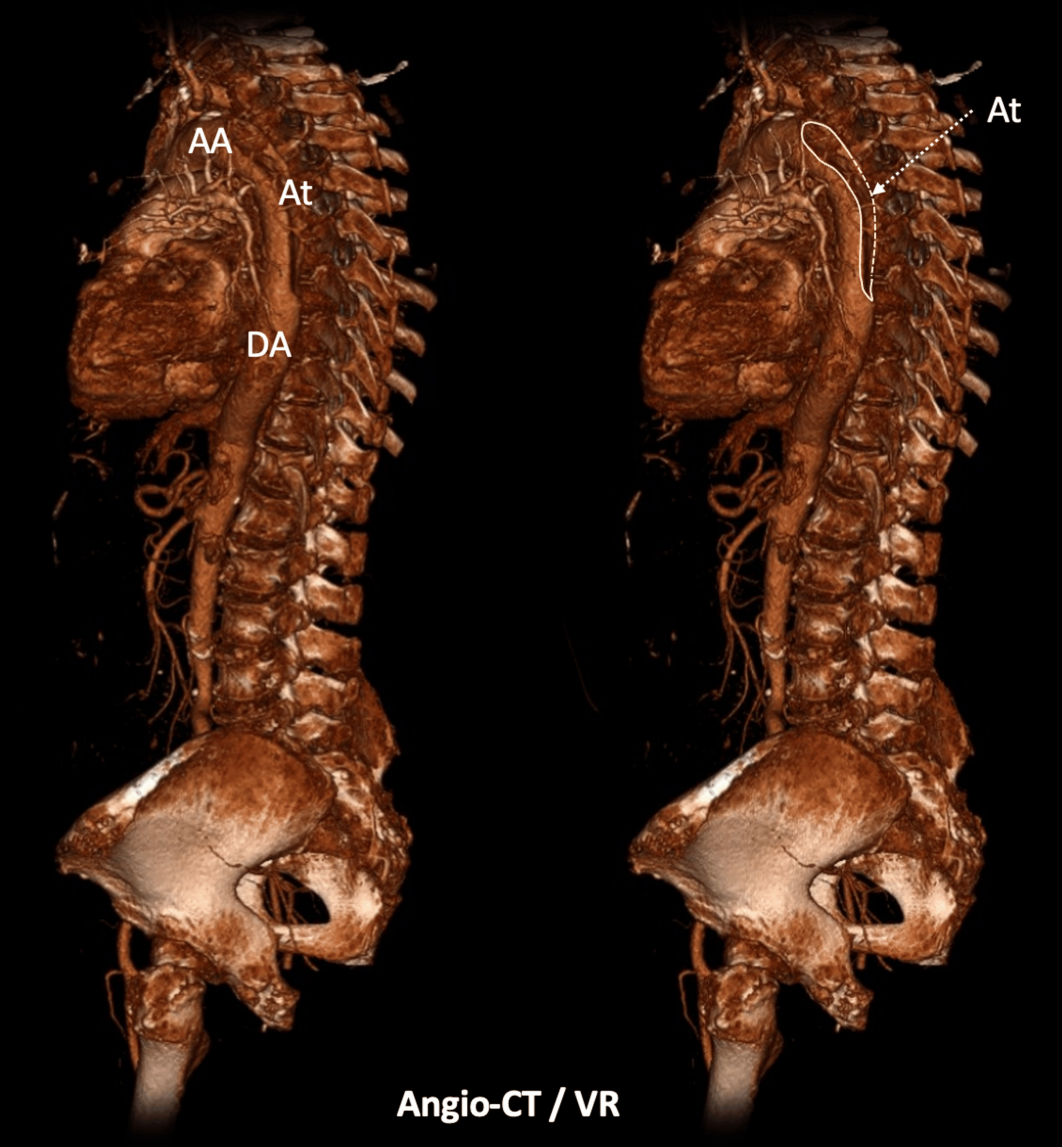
Angio computed tomography - volume rendering AA -Ascending Aorta; At - Aortic Thrombosis; DA - Descending Aorta
